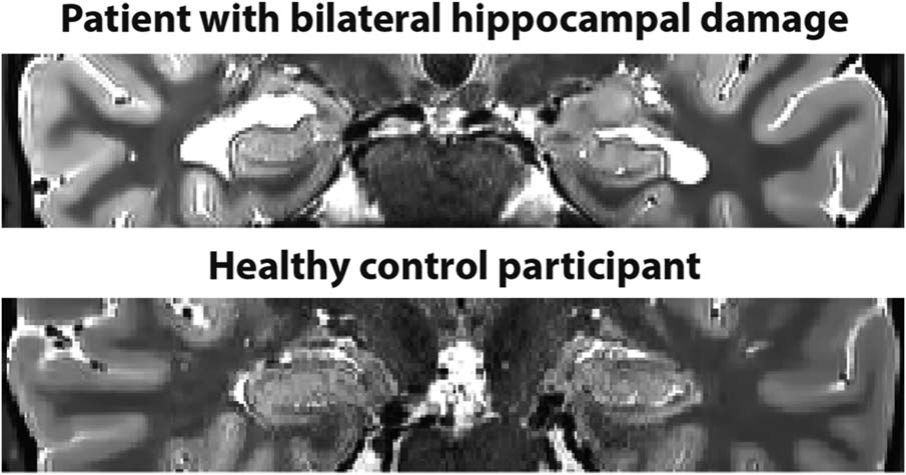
Characterization of hippocampal damage. Example T2‐weighted structural MR image of a patient with selective bilateral hippocampal damage (upper panel) and an age, gender and IQ‐matched healthy control participant (lower panel). Images are displayed in native space corresponding approximately to the position of *y* = −10 in the MNI coordinate system.
